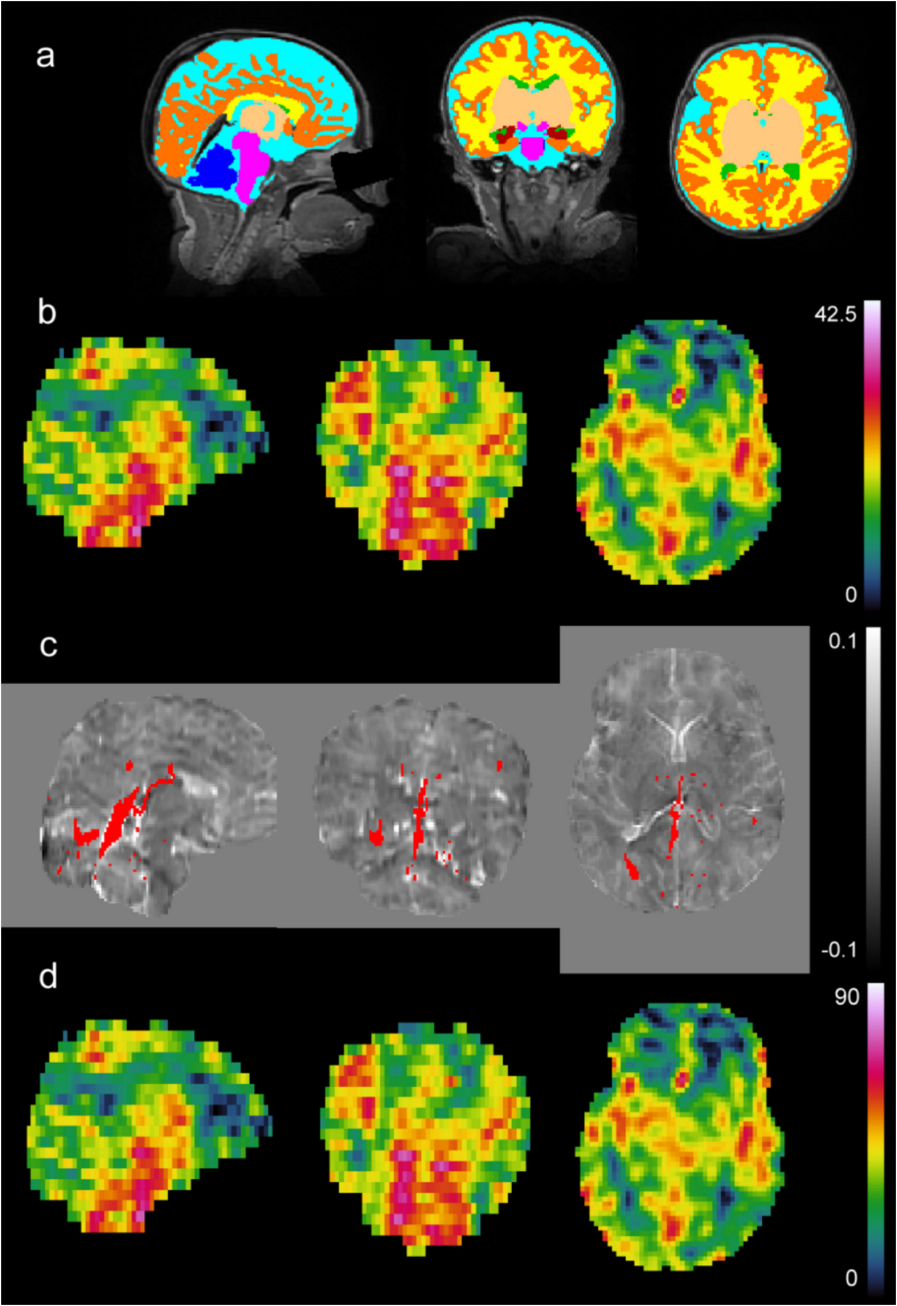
Sample images from a subject of various MRI results. All images show a sagittal, coronal and axial slice from left to right. Panel (A) is a T_2_w image in the background with segmentation overlaid in various colors: dark blue = cerebellum; pink = brainstem; light blue = CSF; yellow = white matter; dark orange = cortical grey matter; green = ventricles; light orange = deep grey matter; dark red = hippocampus and amygdala. Panel (B) is a processed CBF map from 0 to 47.5 mL/100 g/min. Panel (C) is a QSM image in the background from −0.1 to 0.1 ppm susceptibility overlaid with a venous mask in red. D) is a processed CMRO_2_ image from 0 to 93 μmol/100 g/min. Note that panels (B) and (D) look identical as panel (D) is simply panel (B) multiplied by a value determined by CSaO_2_, CSvO_2_, and Hct (see Equation 4). However, this value will be different for every subject.
